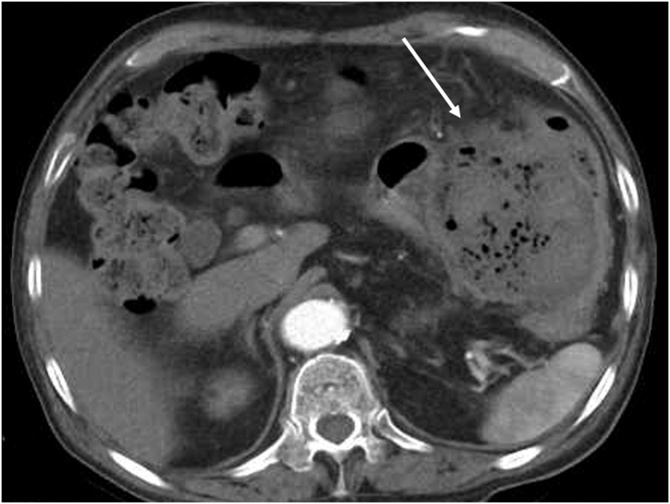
Abdominal contrast-enhanced computed tomography (CT) findings. An air-filled mass (white arrow) measuring 90 mm in diameter.
